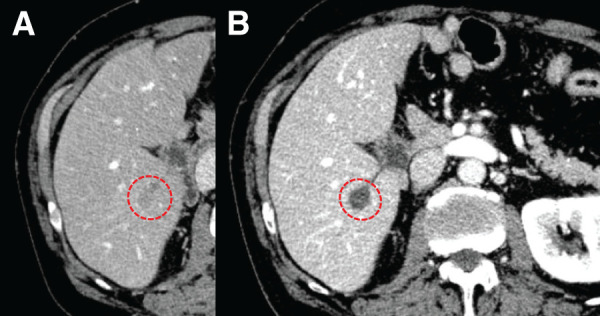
Contrast-enhanced CT images showing liver metastasis from PDAC in segment VI. (A) Contrast-enhanced CT image at the time of liver metastasis diagnosis (dotted circle), 31 months after surgery. (B) Contrast-enhanced CT image showing the liver metastasis (dotted circle) with no significant change in size after systemic chemotherapy. PDAC, pancreatic ductal adenocarcinoma
